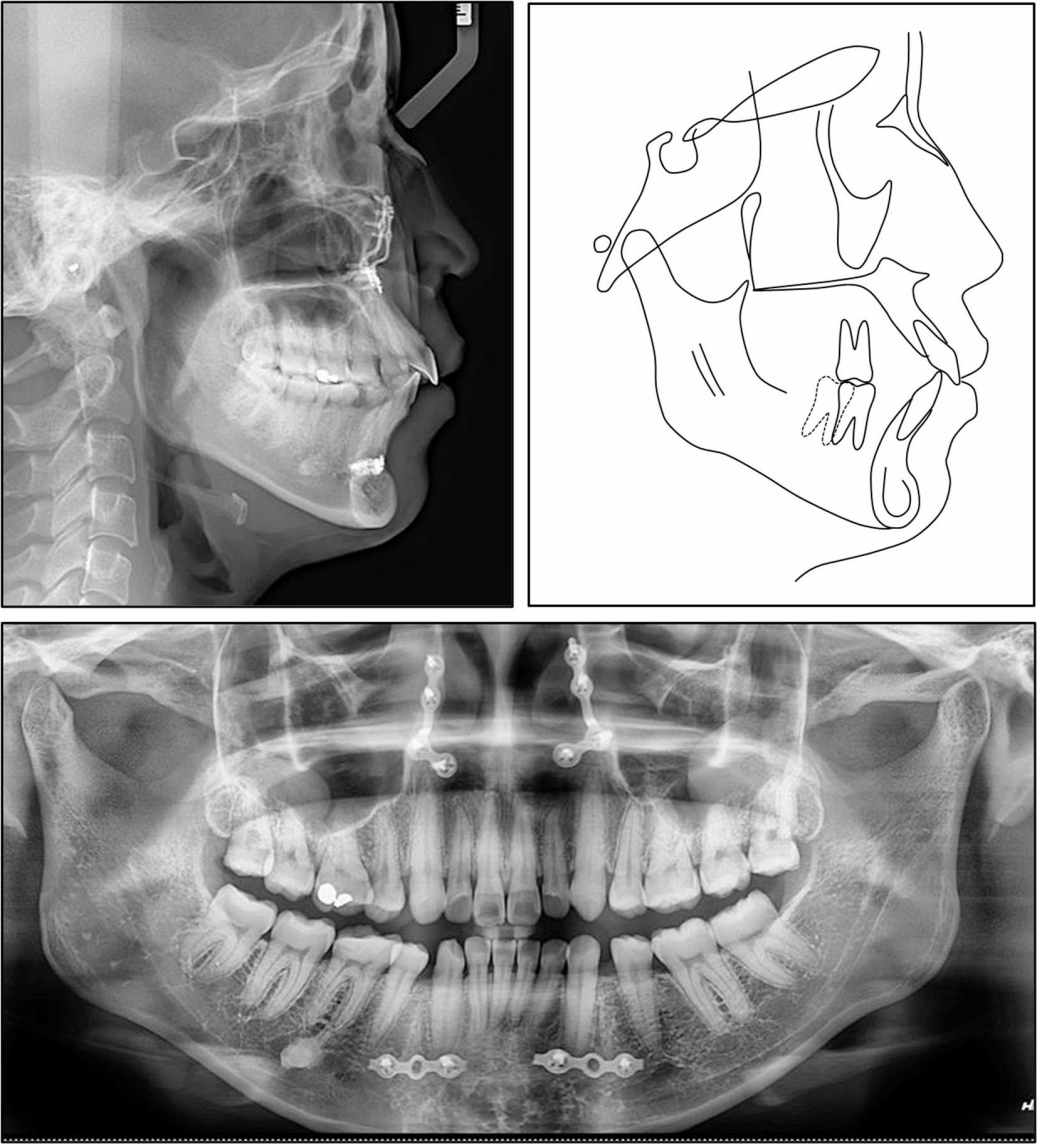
Pre-treatment cephalometric and panoramic radiographs and tracing
